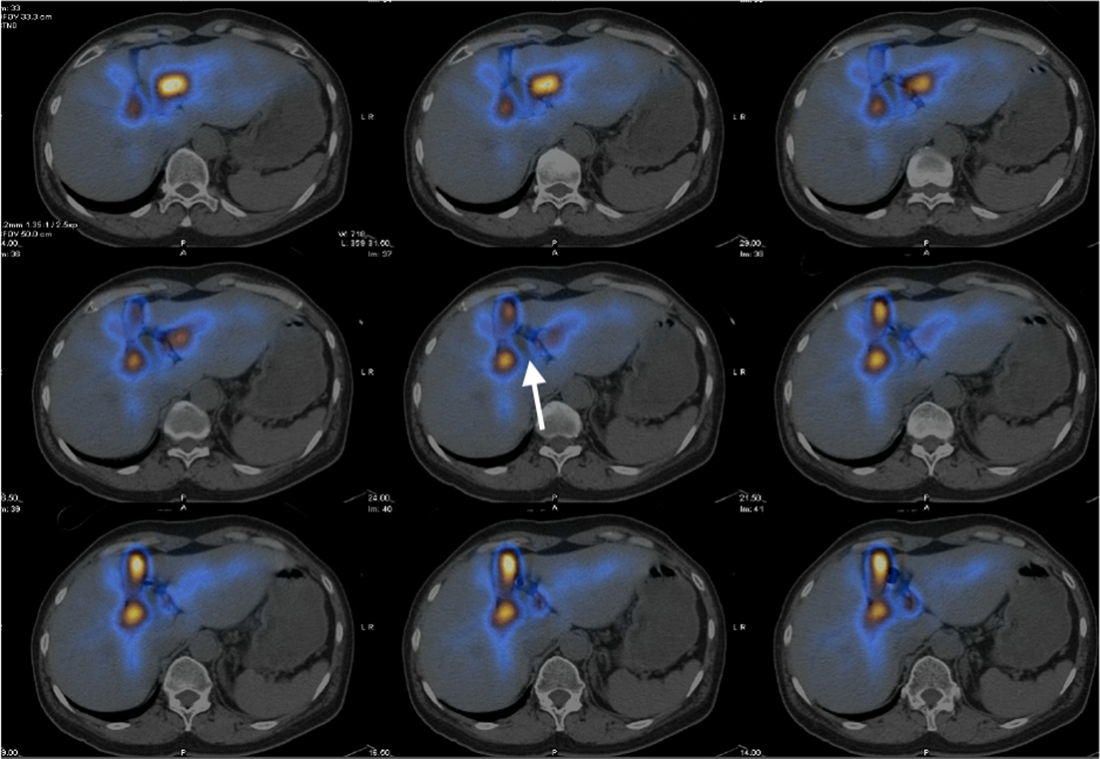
Hepatobiliary scintigraphy with SPECT/CT fusion images showed focal
